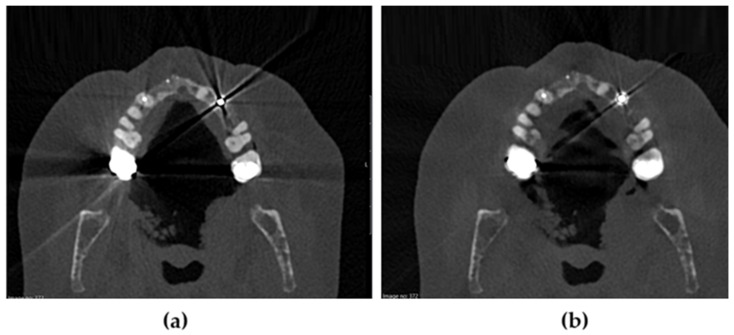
Effect  of iterative metal artifact reduction (IMAR) on maxillofacial photon-counting CT (PCCT). Axial ultra-high-resolution (0.2 mm kernel) PCCT images of the maxillofacial region demonstrating the impact of metal artifact reduction. (a) Image reconstructed without IMAR, showing pronounced streak artifacts originating from dental metallic restorations, partially obscuring adjacent cortical bone and surrounding soft tissues. (b) Image reconstructed with IMAR, demonstrating substantial reduction in streak artifacts and improved delineation of mandibular cortices and adjacent anatomical structures.
